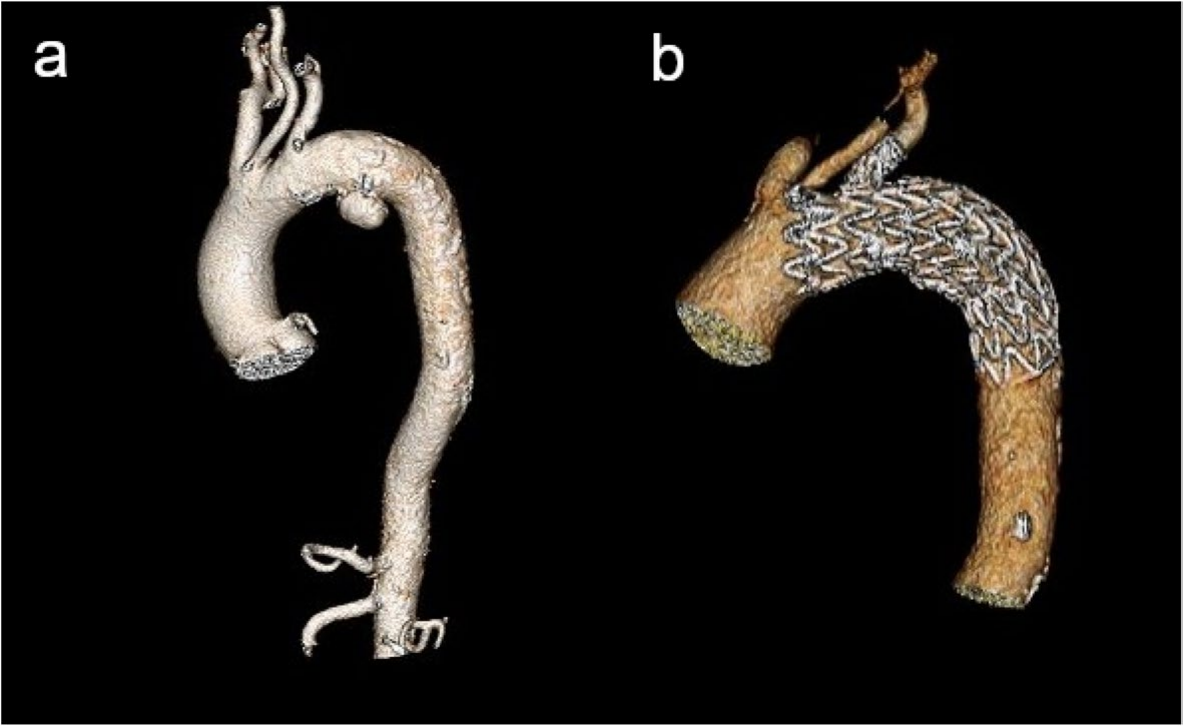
3D reconstructed CTA images of a patient with a descending aorta aneurysm before (a) and after (b) treatment with the single-branched stent graft
